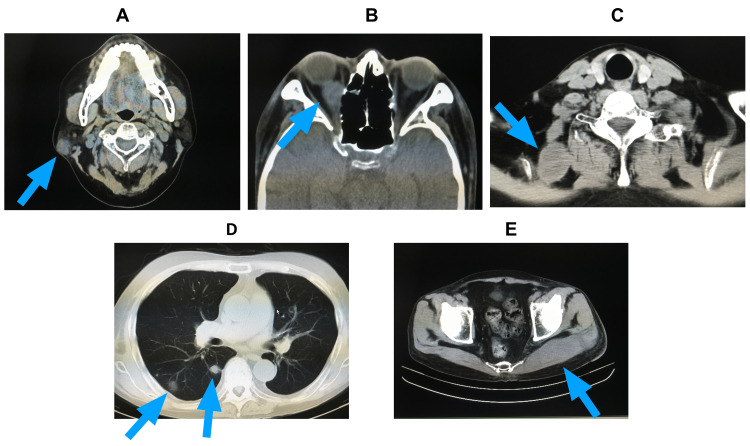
Computed tomography findings on admission. In addition to mass lesions in the right parotid gland area (A) and right orbit (B), the image shows swelling of the right levator scapulae muscle (C) and left gluteal muscle (E). Multiple pulmonary nodules (D) can also be seen.
